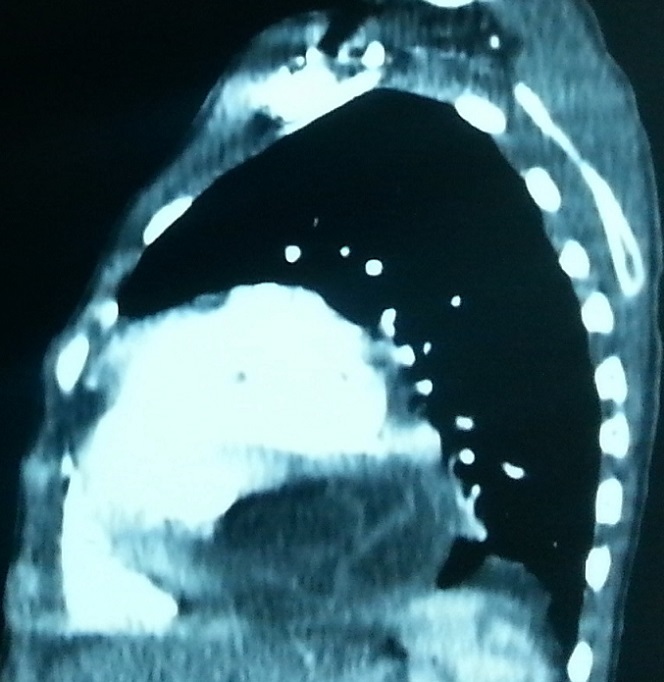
Sagittal CT scan reconstructionsafterinjectionof contrast materialinmediastinalviewshowing the same lesionnext to right cavitieswith a detached layer within it
